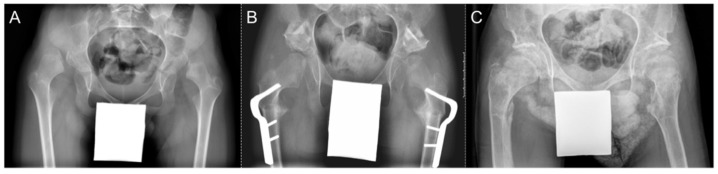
Radiographs of a patient with bilateral CP who underwent bilateral hip reconstruction surgery. (A) Pre-operative. (B) Early post-operative. (C) Late post-operative.
